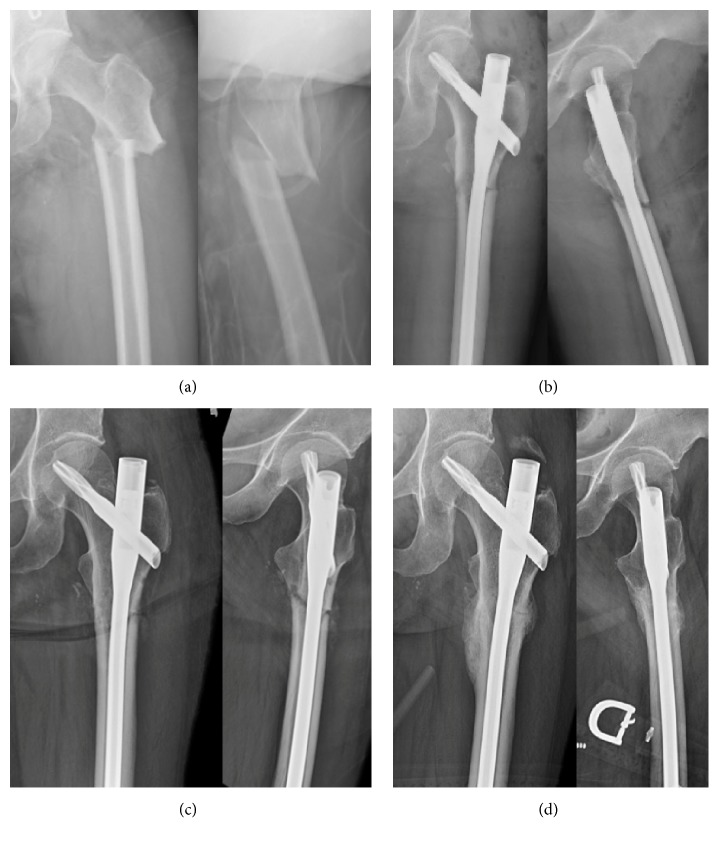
(a) Atypical left femur fracture sustained after a fall from standing in a 58-year-old female with history of bisphosphonate use. (b) She underwent closed reduction and cephalomedullary nailing with a slightly medial start point to induce slight valgus alignment with the trochanteric proximal nail bend. (c) At 6 weeks postoperatively, she denies pain and is ambulating without assistance. Fracture alignment is maintained. (d) At 6 months, she has radiographic union and is back to all activities.
